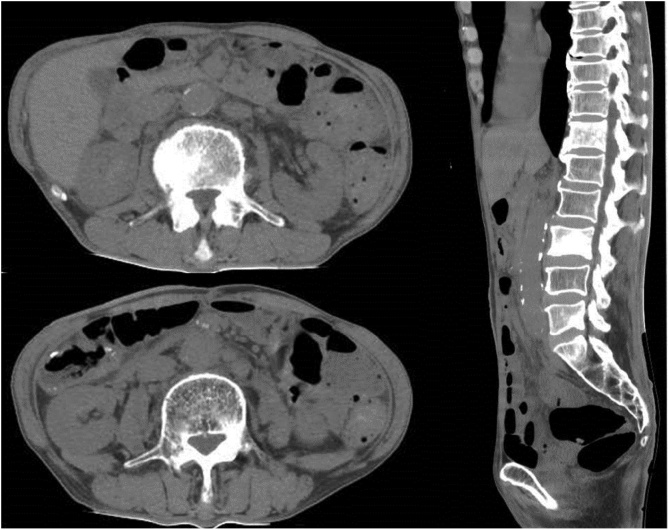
Three months after surgery, abdominal CT revealed metastatic tumor in para-aortic lymph nodes and spine (12th thoracic spine, 3th lumber spine and 5th lumber spine).
